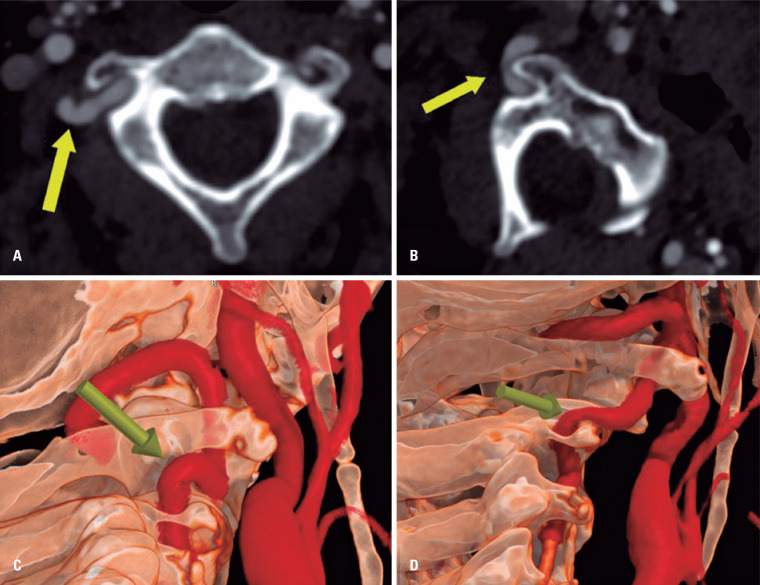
(A) Computed tomography angiography image of axial neutral arterial phase demonstrates a dominant right vertebral artery, with a marked tortuosity in the transition of the V2 to V3 segment, without significant stenosis (arrow in A and C); (B) After left head and neck rotation (position which patient referred symptoms), severe stenosis can be observed in the V2/V3 transition due to compression by the superolateral aspect of the right transverse process of C2; (C) 3D volume rendering image shows the anatomic position without stenosis and (D) after head and neck rotation showing significant stenosis (arrow in B and D)
